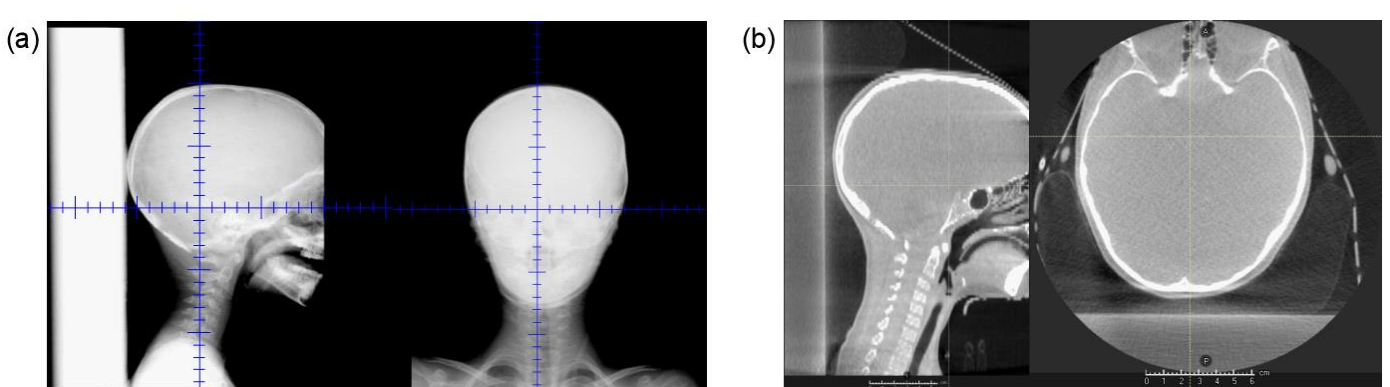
(a) Example (a) orthogonal kV images and (b) CBCT acquired using our on-board proton imaging system from a pediatric patient set up and treated with the RSB present.
